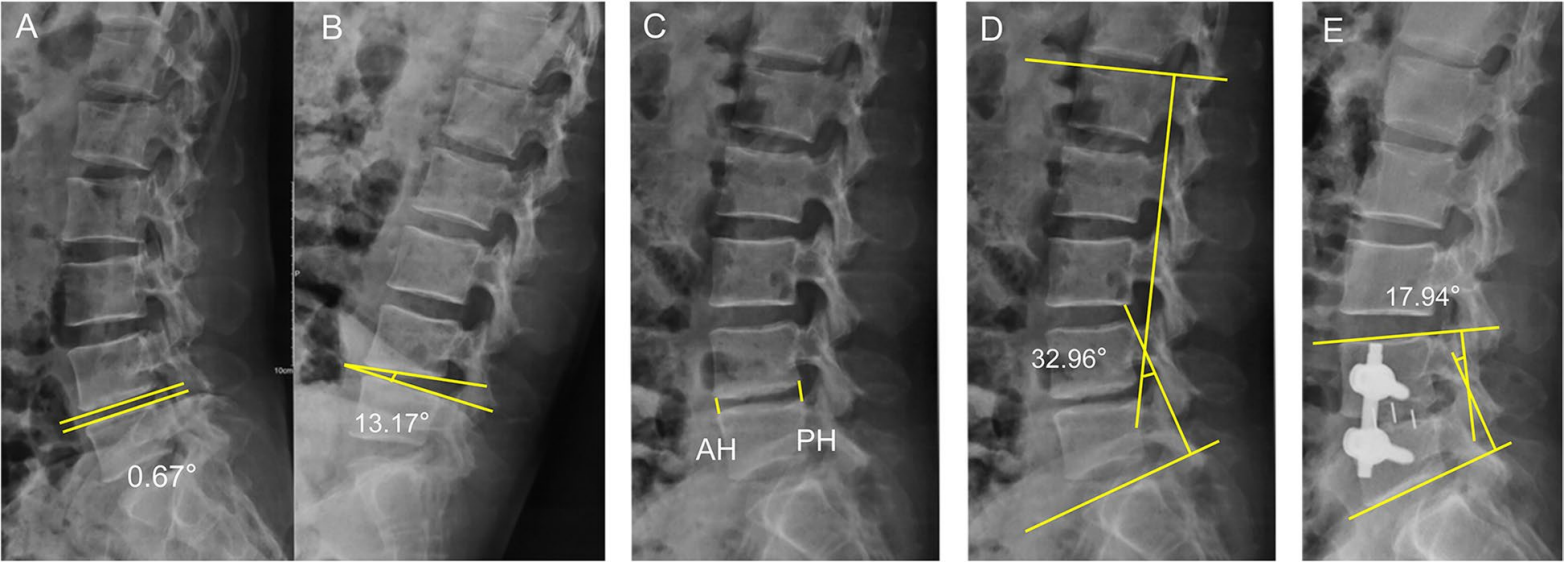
X-ray related index measurement. A, B Preoperative lumbar hyperextension and flexion lateral radiographs showed a change in intervertebral space angulation greater than 10°, which was consistent with the diagnosis of lumbar instability. C Lumbar lateral radiography DH measurement DH = (AH + PH)/2. D Lumbar lateral radiography LA measurement. E FSA measurement on lateral lumbar radiography
